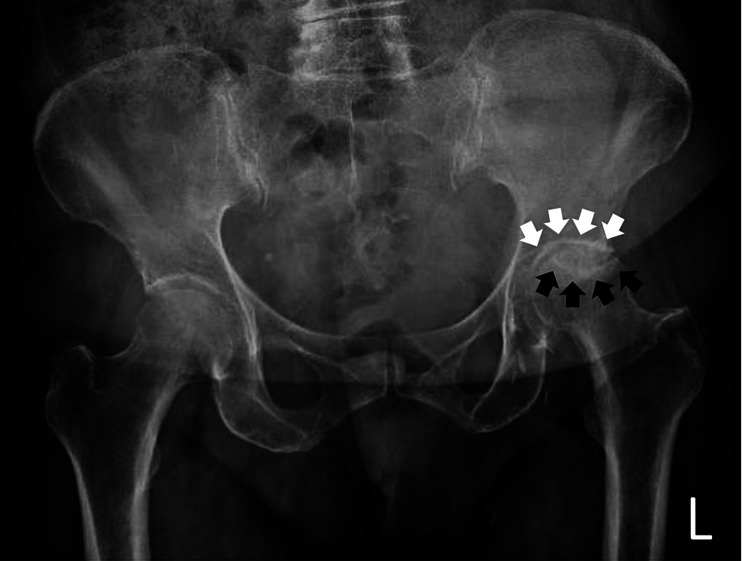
The preoperative radiograph of the patient. Preoperative standard anteroposterior hip and pelvis radiograph depicting the Ficat [3] end-stage avascular necrosis of the left femoral head. Notice the crescent sign (depicted by black arrows) and the sclerotic osteoarthritic border of the acetabulum (depicted by white arrows).
